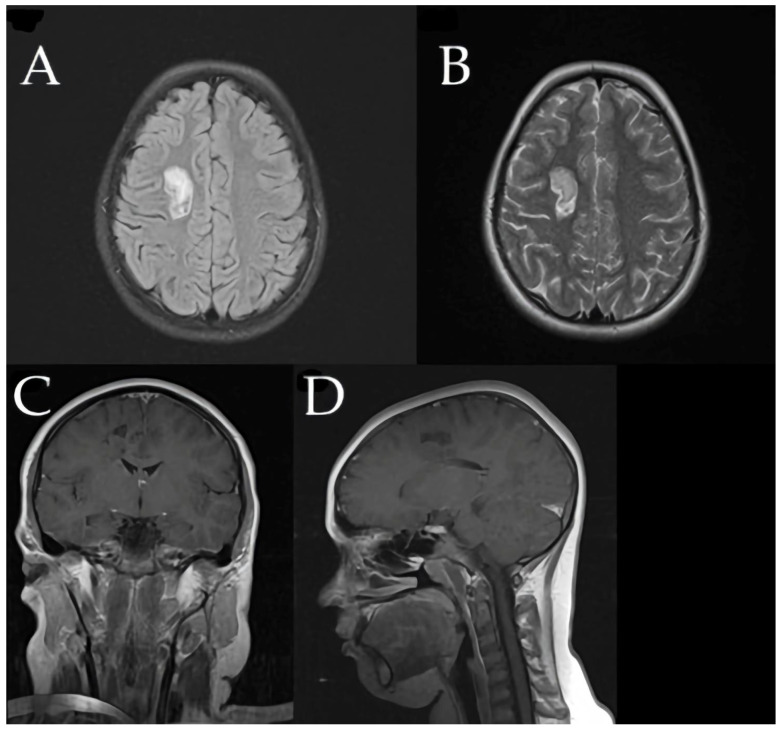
(A–D) One year later, MRI revealed significant decrease in concentric sclerosis 18 × 14 × 12.2 mm, with no new demyelinating lesions. Axial FLAIR (A) and axial T2-weighted (B) images demonstrate reduced lesion size and diminished hyperintense signal intensity. Post-contrast coronal (C) and sagittal (D) T1-weighted images confirm absence of active inflammation or enhancement, highlighting lesion stability and significant regression from initial presentation.
